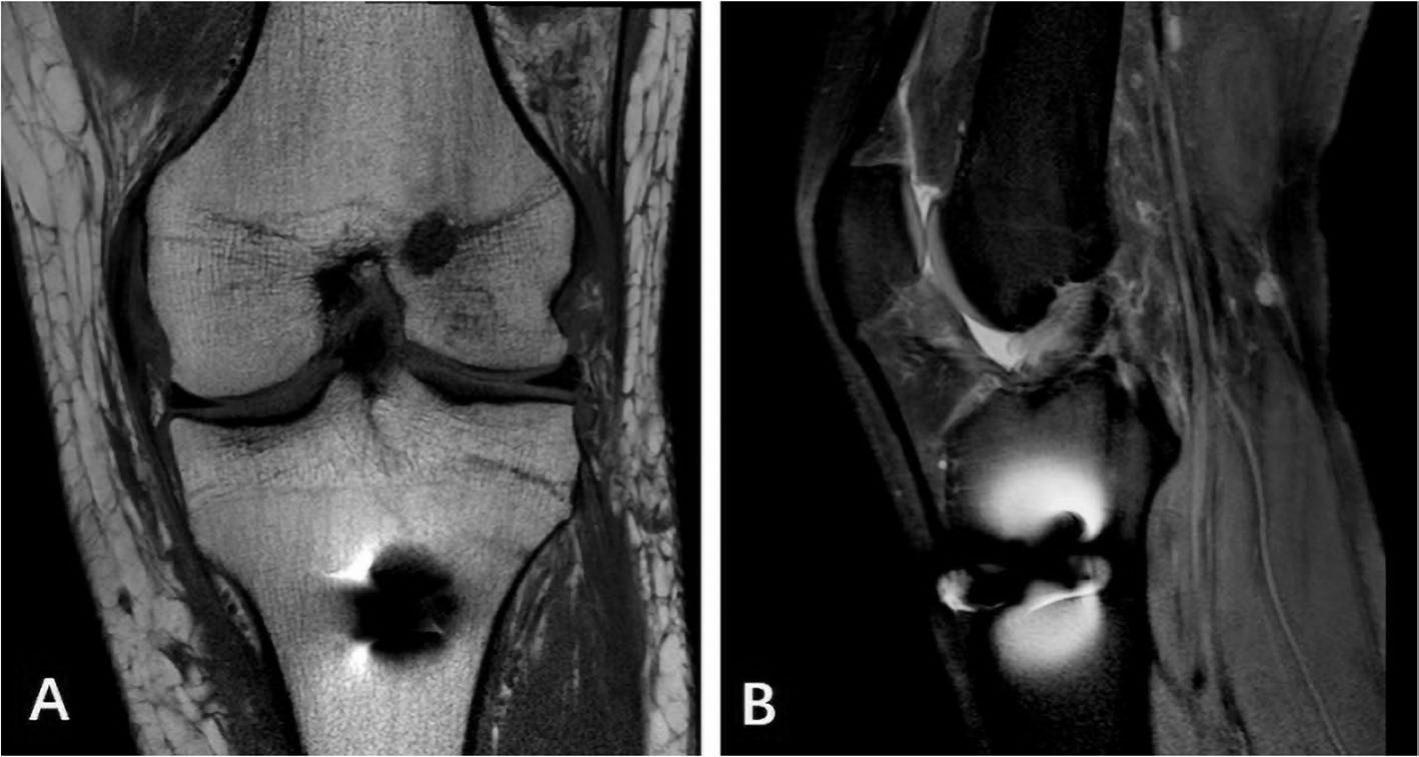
Postoperative follow-up of 26-year-old male patients with ACL reconstruction: a Reexamination of magnetic resonance imaging one week after operation, the intercondylar notch width index (NWI) was 0.19; b half a year after operation, MRI showed that reconstruction of ACL impingement and peripheral synovial hyperplasia
